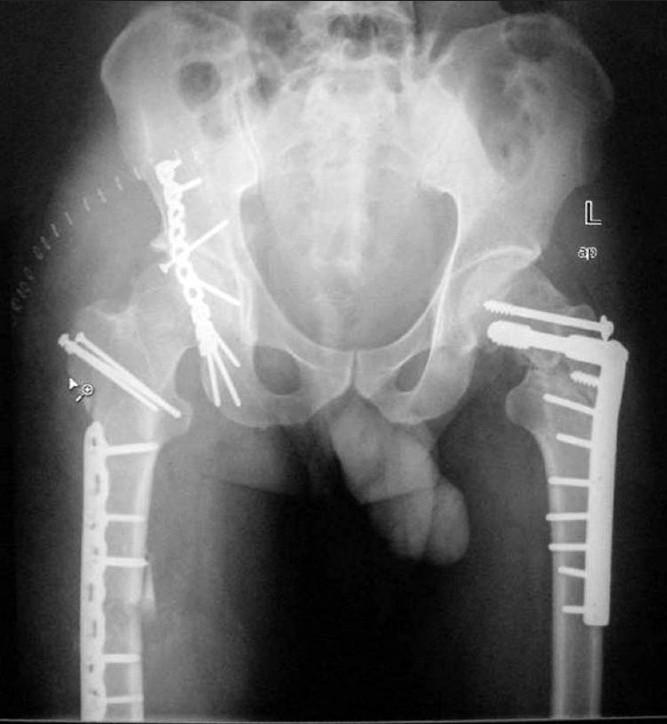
Postoperative radiograph of the pelvis with both hips and thighs (an anteroposterior view) showing internal fixation of all the injuries
